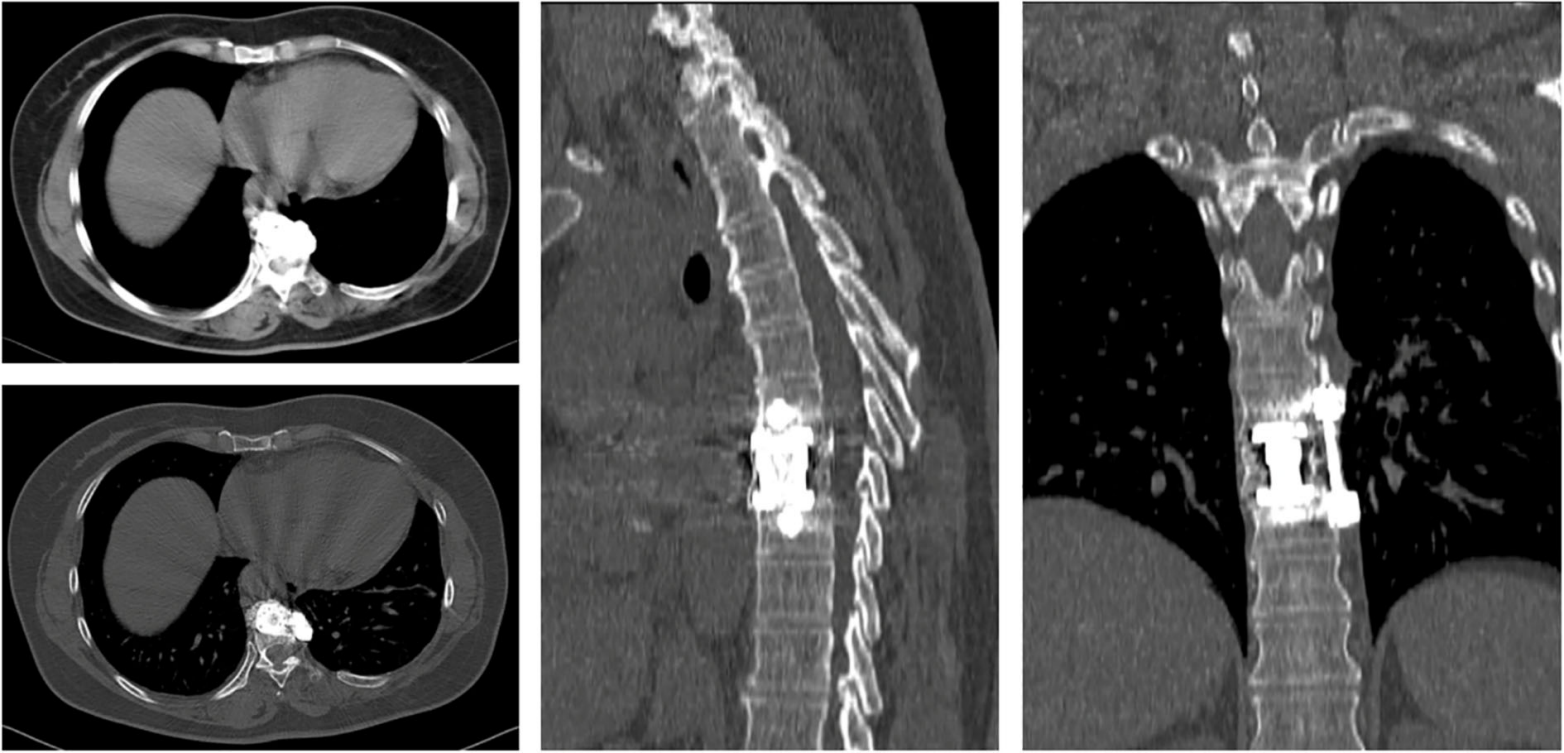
CT imaging reveals no evidence of recurrence during the 1-year follow-up period, with internal fixation in place.
